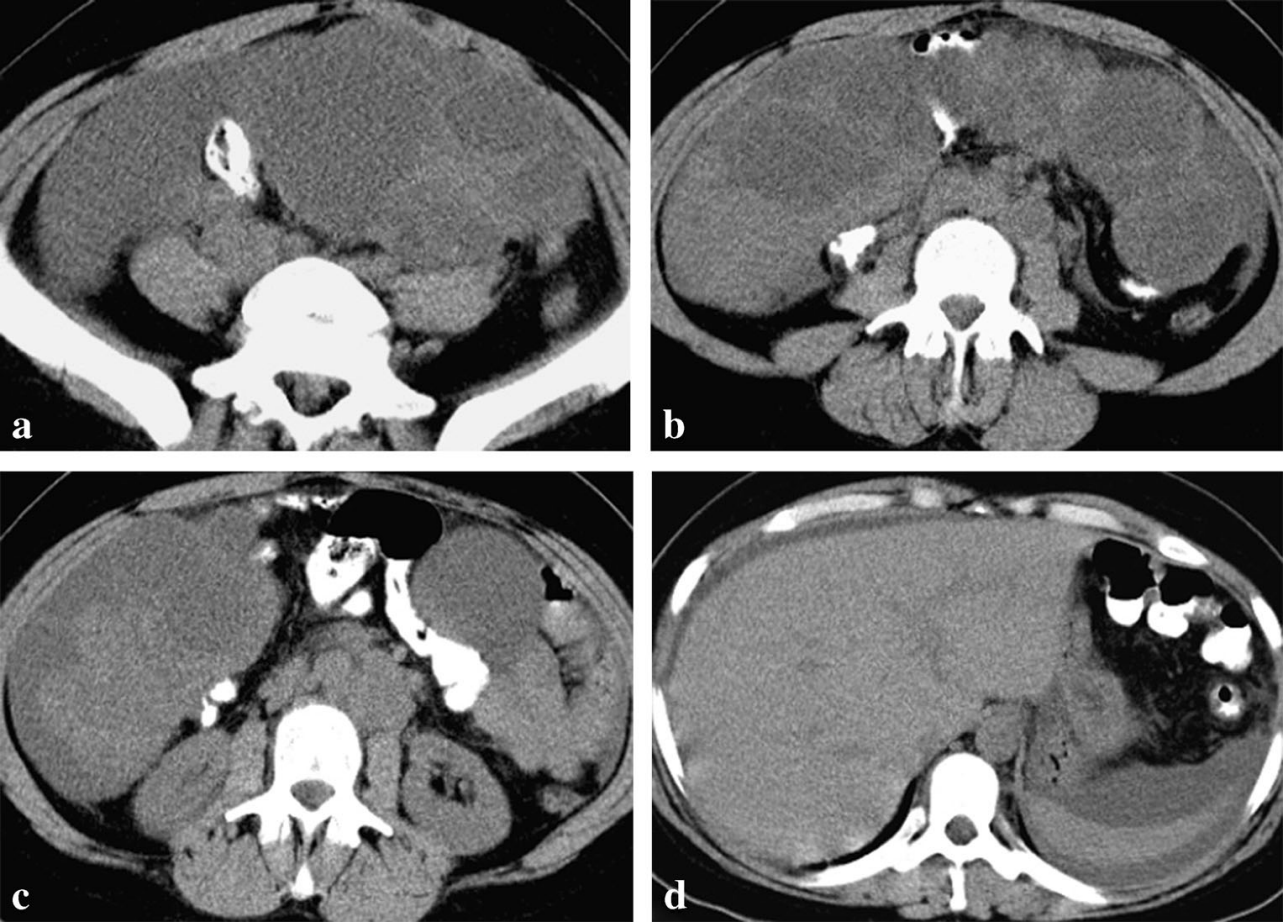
a-d. Bilateral undifferentiated ovarian carcinomas in a 34-year-old BRCA1-mutation carrier woman. CT scan shows a large multiloculated cystic left adnexal mass with thick septa (a). A right adnexal mass, similar to that one described in image a, but with a more prominent solid component, was also present (b). Left para-aortic lymph node metastases are also present (c). Moderate ascites is evident in all images but there was no pleural effusion (d). Pathologic examination ruled out ovarian capsule and tubal invasion
